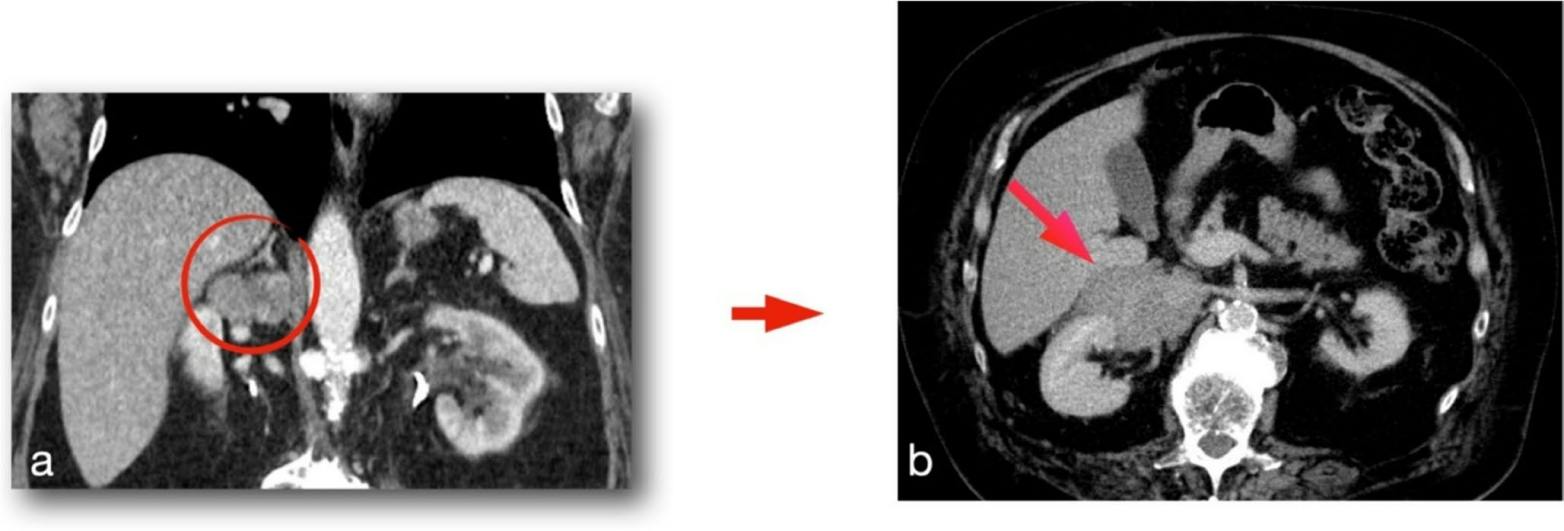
CT scan showing tumor prior to partial adrenalectomy (a) for ACC; recurrence developed after 12 months (b)
